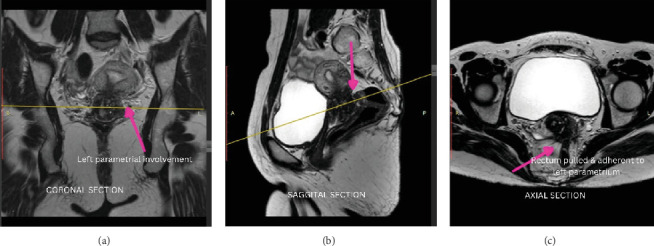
T2-weighted MR images in the (a) coronal, (b) sagittal, and (c) axial planes depicting an endometriosis nodule in the left parametrium involving the rectum and vagina.
